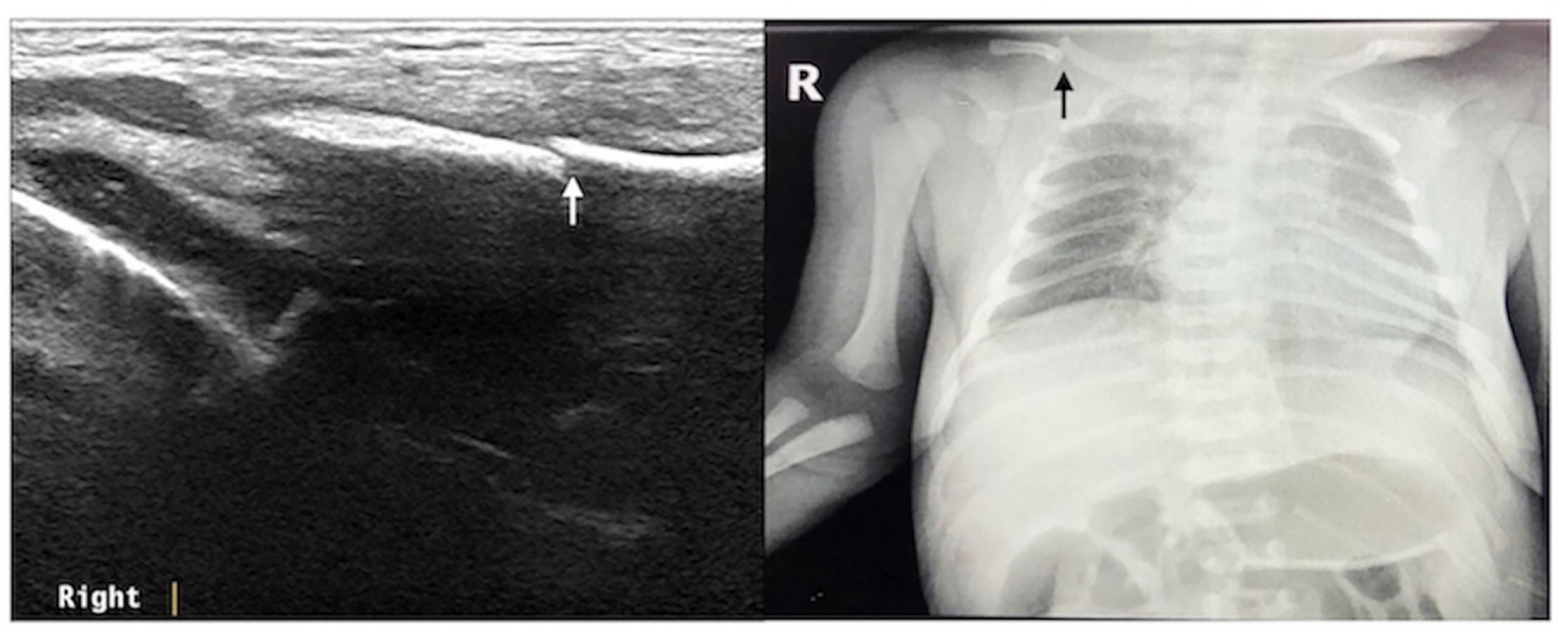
Right clavicle fracture. The infant was G_2_P_1_ with a gestational age of 39^+1^ weeks, vaginal delivery, and birth weight of 4,140 g. The patient was admitted to the hospital 3 h after birth due to intrauterine pneumonia. Physical examination showed that the bilateral clavicles were asymmetrical and that the left side was smooth, while the right side had obvious bone rubbing. Ultrasound revealed interrupted continuity of the cortical bone of the right clavicle and broken end formation and dislocation. The fracture of the clavicle was confirmed by chest X-ray examination.
